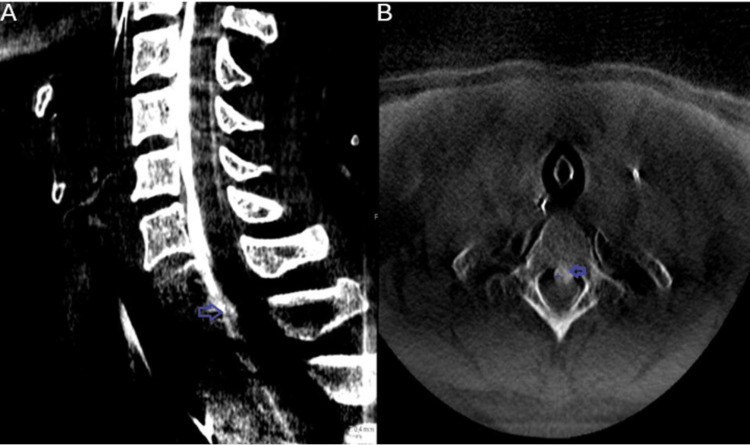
Cervical CT myelogram (A) Sagittal CT myelogram demonstrates a rostral osteophyte at the C7-T1 level with a gradient of myelographic contrast indicating a CSF leak (blue arrowheads). (B) Axial CT myelogram demonstrates the sharp, posteriorly directed osteophyte anterior to the spinal cord; the blue arrowheads show the epidural contrast collection ventral to the spinal cord. CT: computed tomography; CSF: cerebrospinal fluid
